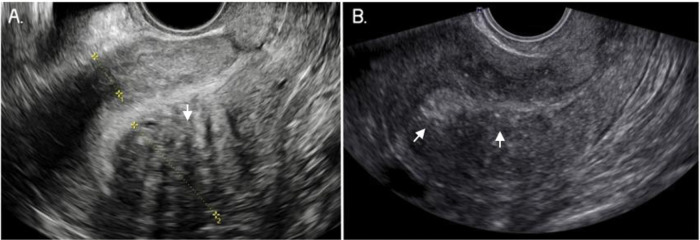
Two-dimensional ultrasound imaging of a uterus in a longitudinal section showing typical sonographic features of adenomyosis. A. Note the asymmetry of the myometrial wall (posterior wall thicker than anterior wall) and the heterogeneous myometrium with hypoechoic linear striae (arrows). B. Poorly defined junctional zone (arrows).
